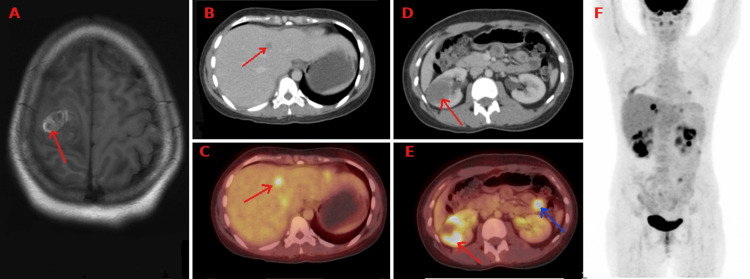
Cross-sectional MRI image (A) reveals a well-circumscribed lesion (red arrow) in the right high frontoparietal lobe within the evidence of an intralesional hemorrhagic signal. Cross-sectional CT image (B) and fused PET CT image (C) reveal FDG avid hypodense metastatic lesion in segment IV of the liver (red arrow). Cross-sectional CT image (D) and fused PET CT image (E) reveal FDG avid hypodense metastatic lesion involving the right kidney (red arrow). Images D and E also reveal FDG avid hypodense metastatic lesion involving the jejunal bowel loop (blue arrow). Maximum intensity projection (MIP) image F reveals increased foci of FDG uptake in the liver, bilateral kidneys, bowel loops, and lung regions corresponding to metastatic lesions.
